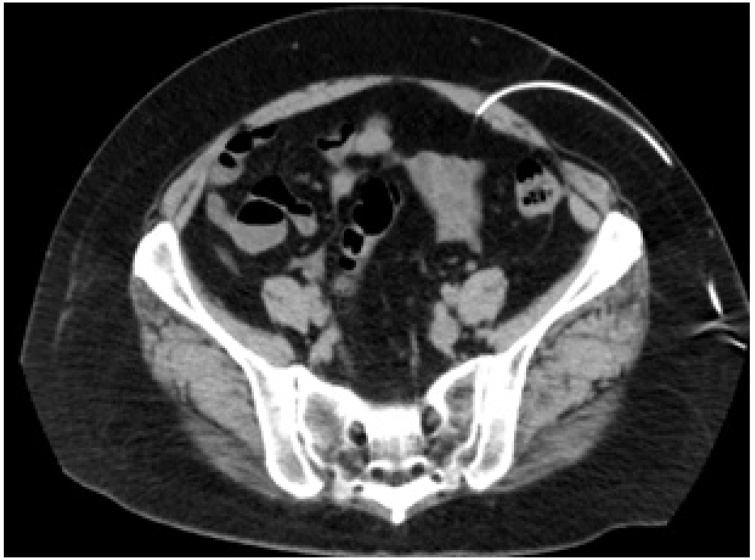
CT image of the route of the shunt. The shunt tube ran through the left flank to the abdominal cavity.
